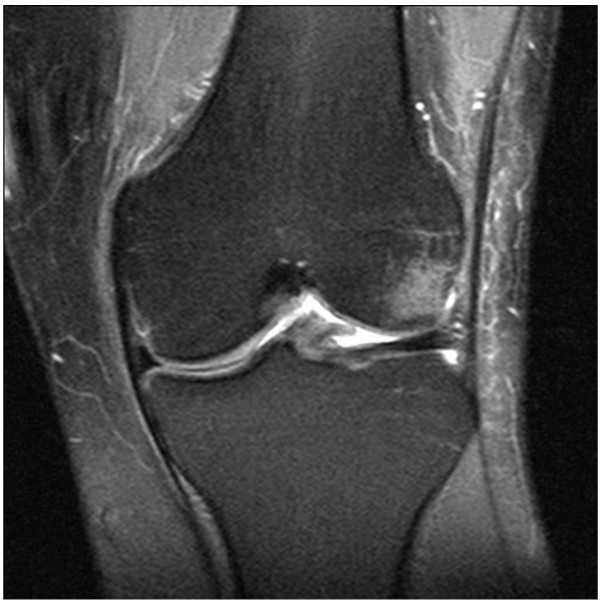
Coronary MRI sequence. Non-appearance of MFH before ACL rupture in 2006. Bone bruise in the lateral femoral condyle.
